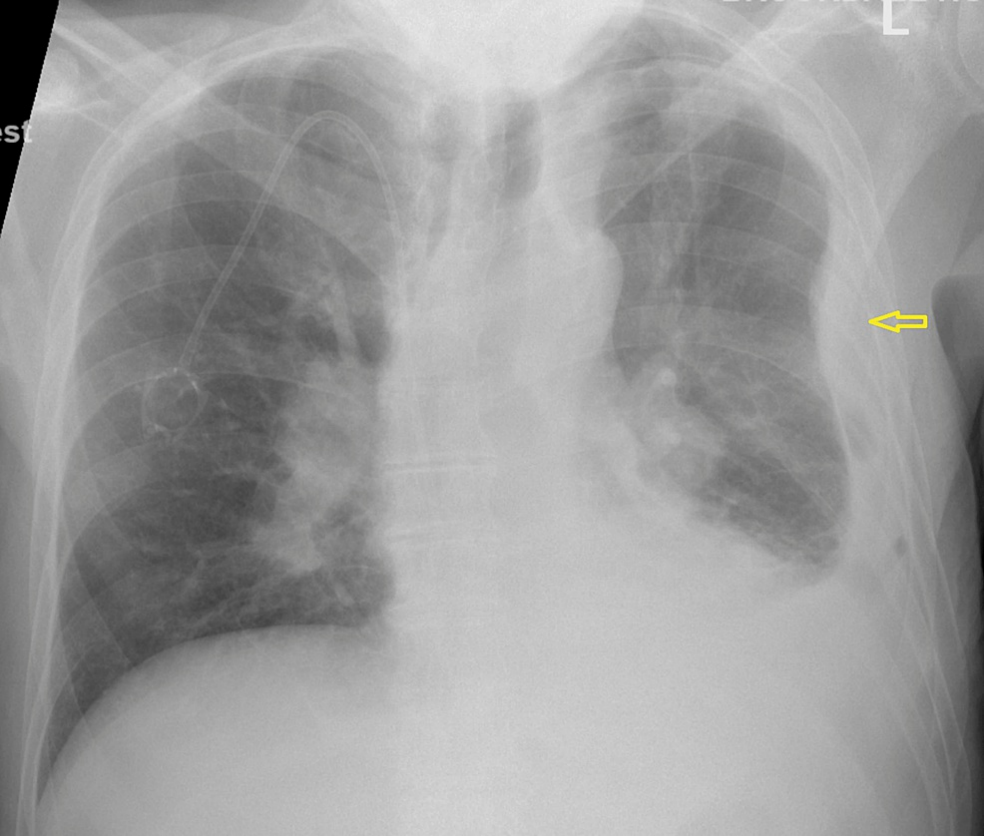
Chest radiograph demonstrating “trapped lung” - a complication of loculated pleural effusion
